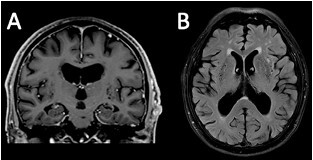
Magnetic resonance imaging (MRI). Coronal T1-weighted image shows no focal cortical atrophy, with bilateral medial temporal atrophy (MTA) score of 2 and ERICA score of 1, consistent with age-related changes. Axial T2-FLAIR image reveals marked white matter hyperintensities, classified as grade 3 on the Fazekas scale, and enlarged perivascular spaces in the basal ganglia and corona radiata, raising the differential of chronic lacunar lesions, suggesting associated microangiopathy.
